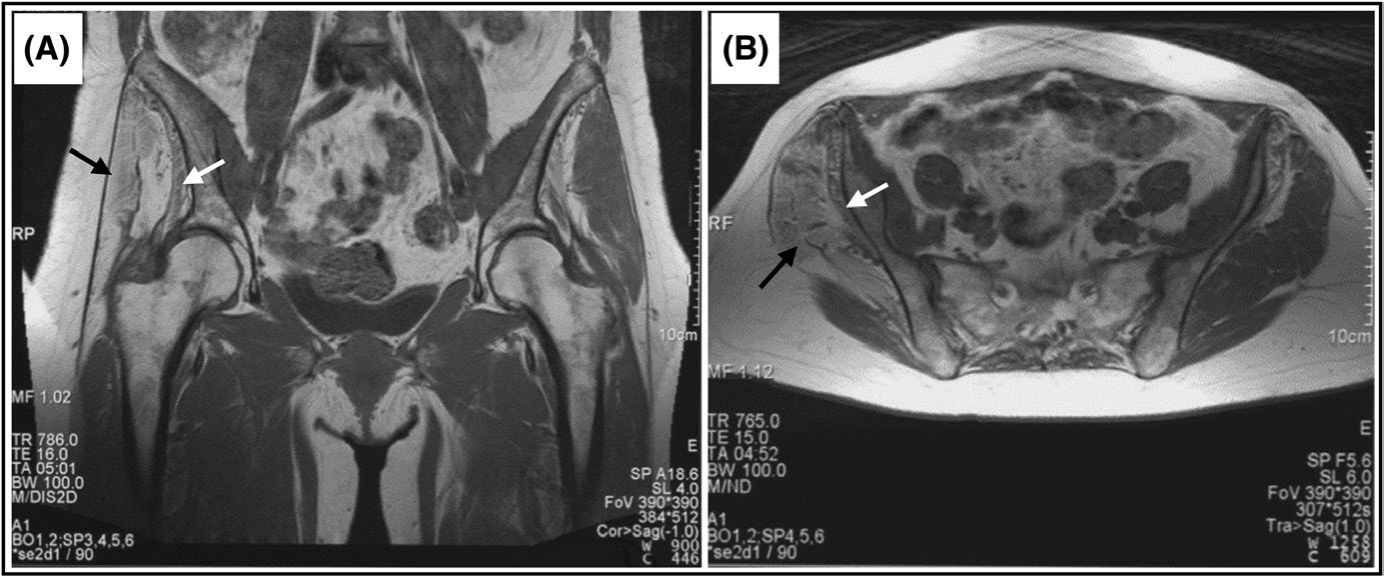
Magnetic resonance imaging of the pelvis. A, Coronal view and B, Axial view depicting diffuse fatty infiltration of the right gluteus medius and minimus. Gluteus medius, black arrows; gluteus minimus, white arrows
